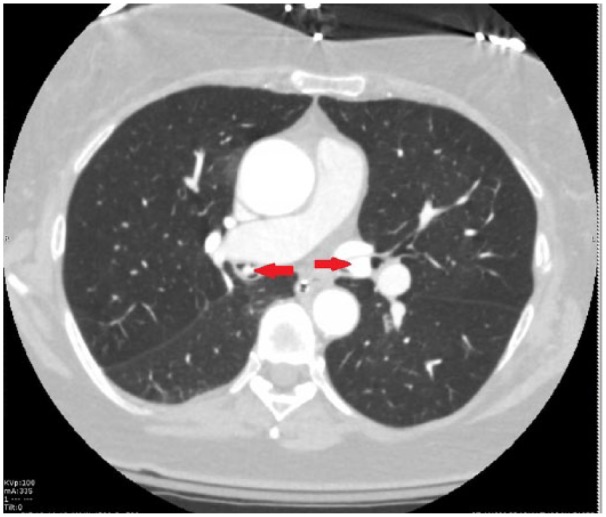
Axial view of computed tomography scan of thorax showing calcified foreign objects (red arrows) in the left and right main bronchus.
